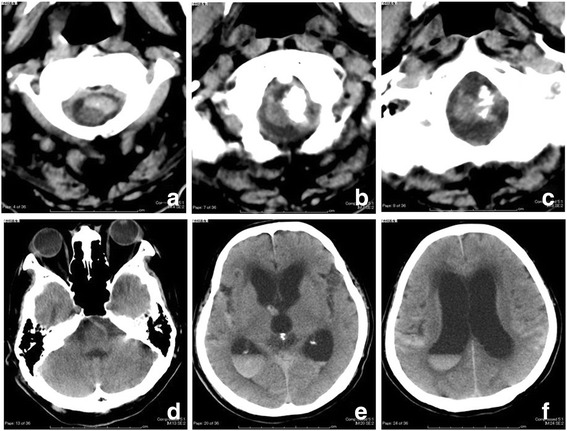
Repeated computed tomography demonstrating hemorrhaging at the ventral side of the cervical calcified lesion (a-c), as well as subarachnoid, intraventricular hemorrhaging and acute hydrocephalus (d-f)
